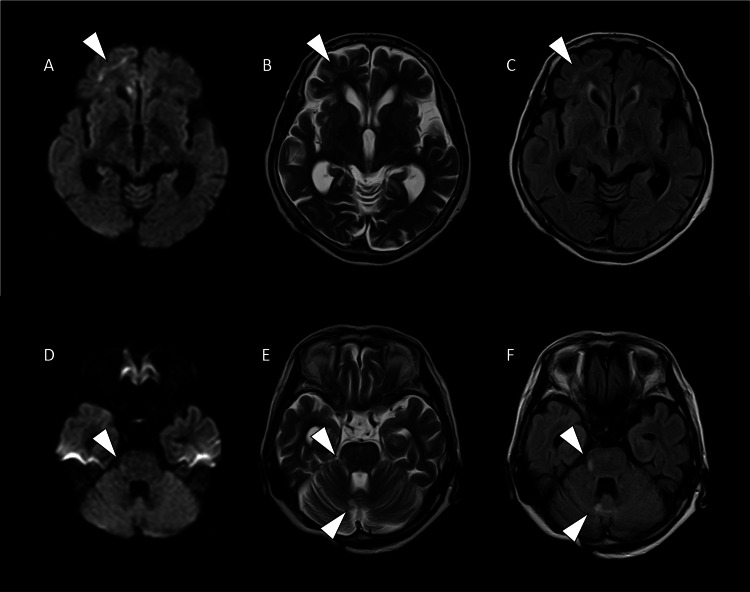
Brain MRI at initial presentation DWI: diffusion-weighted imaging; T2WI: T2-weighted imaging; FLAIR: fluid attenuated inversion recovery DWI (A,D) showing a ribbonlike hyperintensity (arrowhead) at the right frontal corticomedullary junction and hyperintense lesions (arrowhead) in the middle cerebellar peduncle. T2WI (B,E) and FLAIR (C,F) images showing hyperintense lesions (arrowhead) in the right frontal corticomedullary junction, middle cerebellar peduncle, and paravermian cerebellar region
